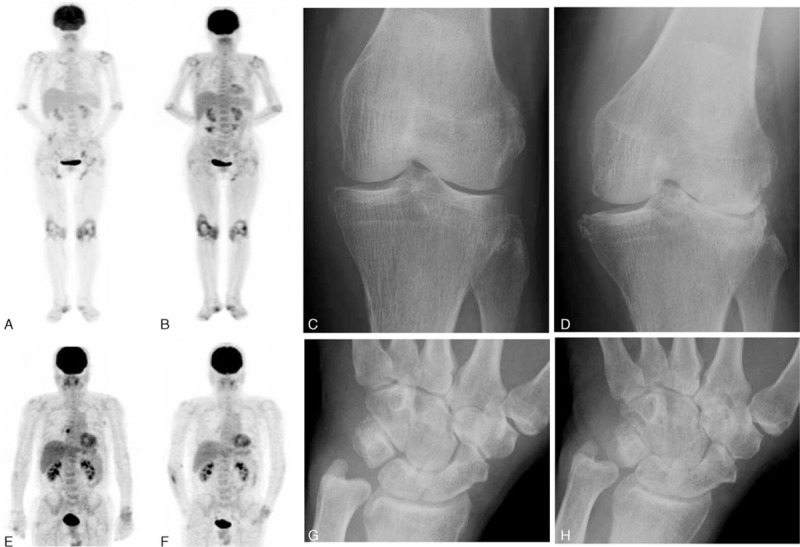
Typical ^18^F-FDG PET/CT images and radiographs with a progression of joint destruction. A 63-year-old woman with a 32-year history of RA treated with TCZ therapy. Anterior image obtained on ^18^F-FDG PET/CT at baseline (A) and 6 months after the initiation of biological therapy (B). The Larsen grade in the left knee joint progressed from grade III (C) to grade IV (D). A 70-year-old man with a 3-year history of RA treated with IFX therapy. ^18^F-FDG PET/CT findings at baseline and 6 months after treatment (E, F). The Larsen grade in the left wrist progressed from grade II to grade III (G, H). Progression of joint destruction was shown in the joints with high FDG uptake at baseline and 6 months. FDG-PET/CT = fluorodeoxyglucose positron emission tomography combined with computed tomography, RA = rheumatoid arthritis, TCZ = tocilizumab.
